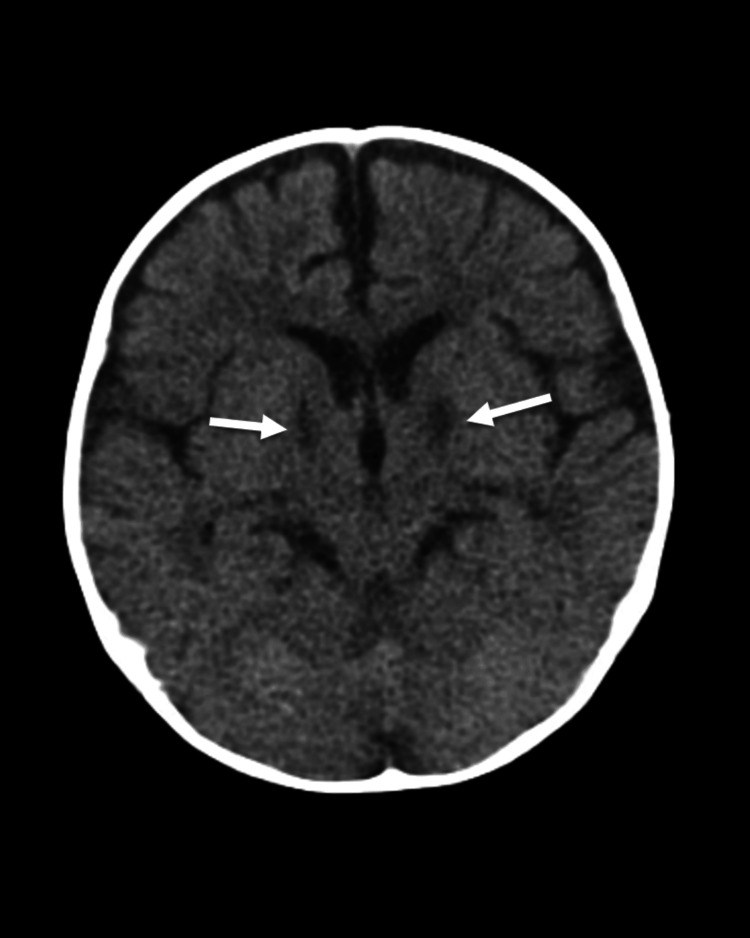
Repeat axial non-contrast CT showing persistent symmetric hypodense foci at the inferior basal ganglia, approximately the genu of the internal capsule, consistent with the MRI appearance. These could be possible small infarcts.
